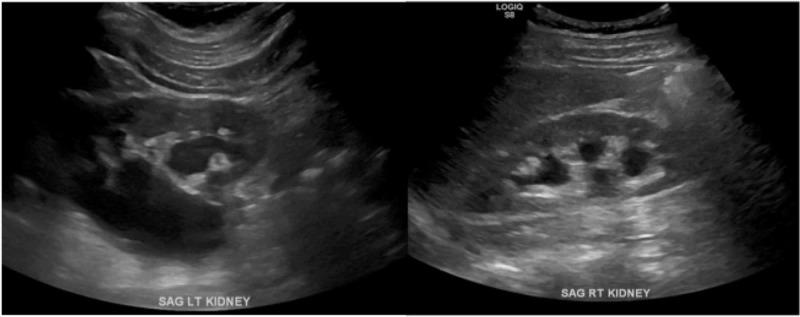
Renal ultrasound showing bilateral hydronephrosis SAG LT KIDNEY: shows a sagittal view of the left kidney; SAG RT KIDNEY: shows a sagittal view of the right kidney.
